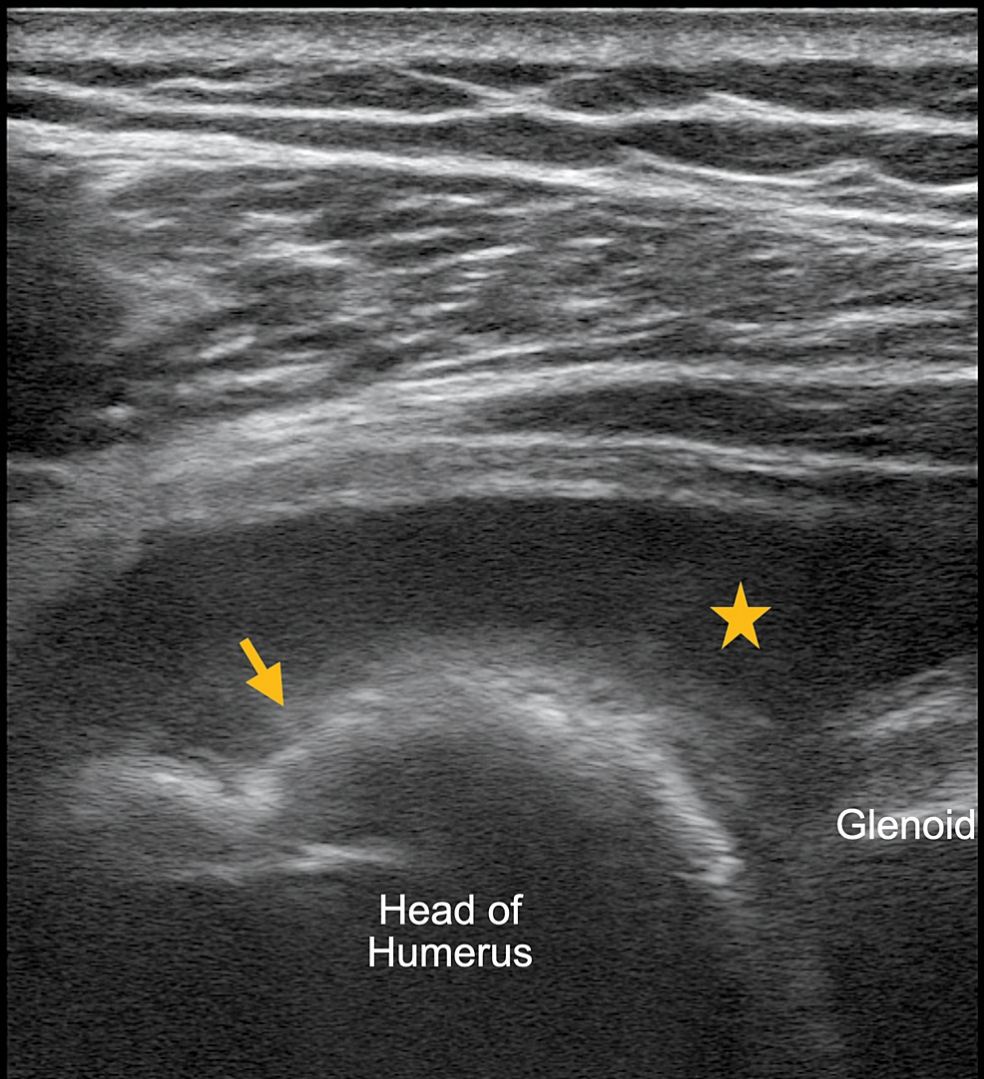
Ultrasound image of the left shoulder using a high-frequency linear probe revealed cortical erosion of the humeral head (arrow) with a joint effusion (star).
